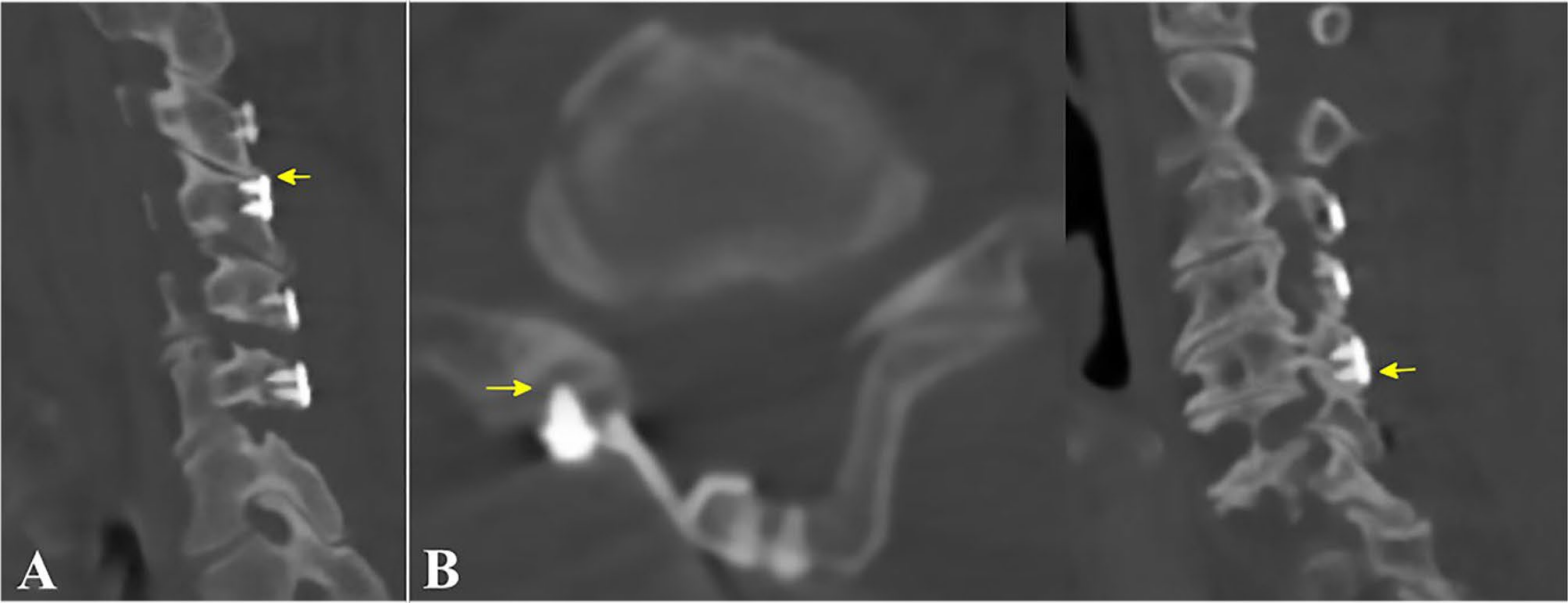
Inappropriate plate positioning. (A) Cranially located mini-plate causes impingement with the lateral mass of the proximal adjacent level (arrow). (B) Screw facet joint violation is detected in axial and sagittal computed tomography images when the mini-plate is located too caudally (arrows).
